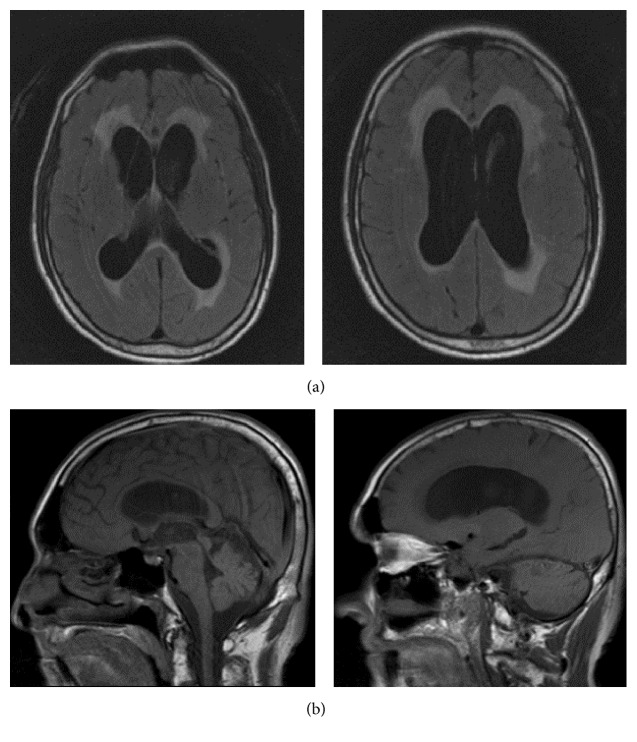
MRI FLAIR axial (a) and sagittal (b) notable for communicating hydrocephalus.
